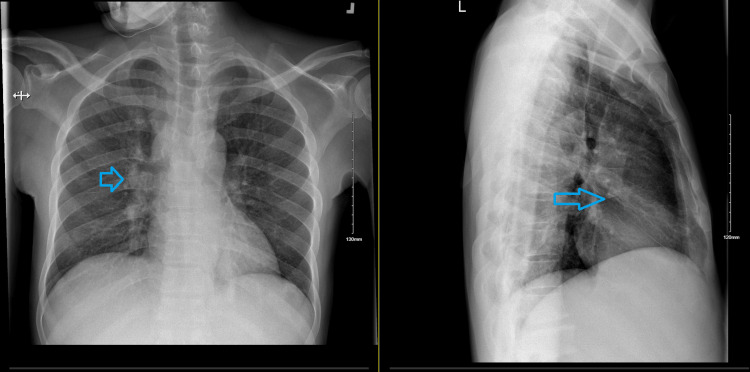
CXR (anteroposterior view - left and lateral view - right) Blue arrows: faint airspace opacity seen probably in the right middle lobe, better seen on the lateral view CXR: Chest X-ray
